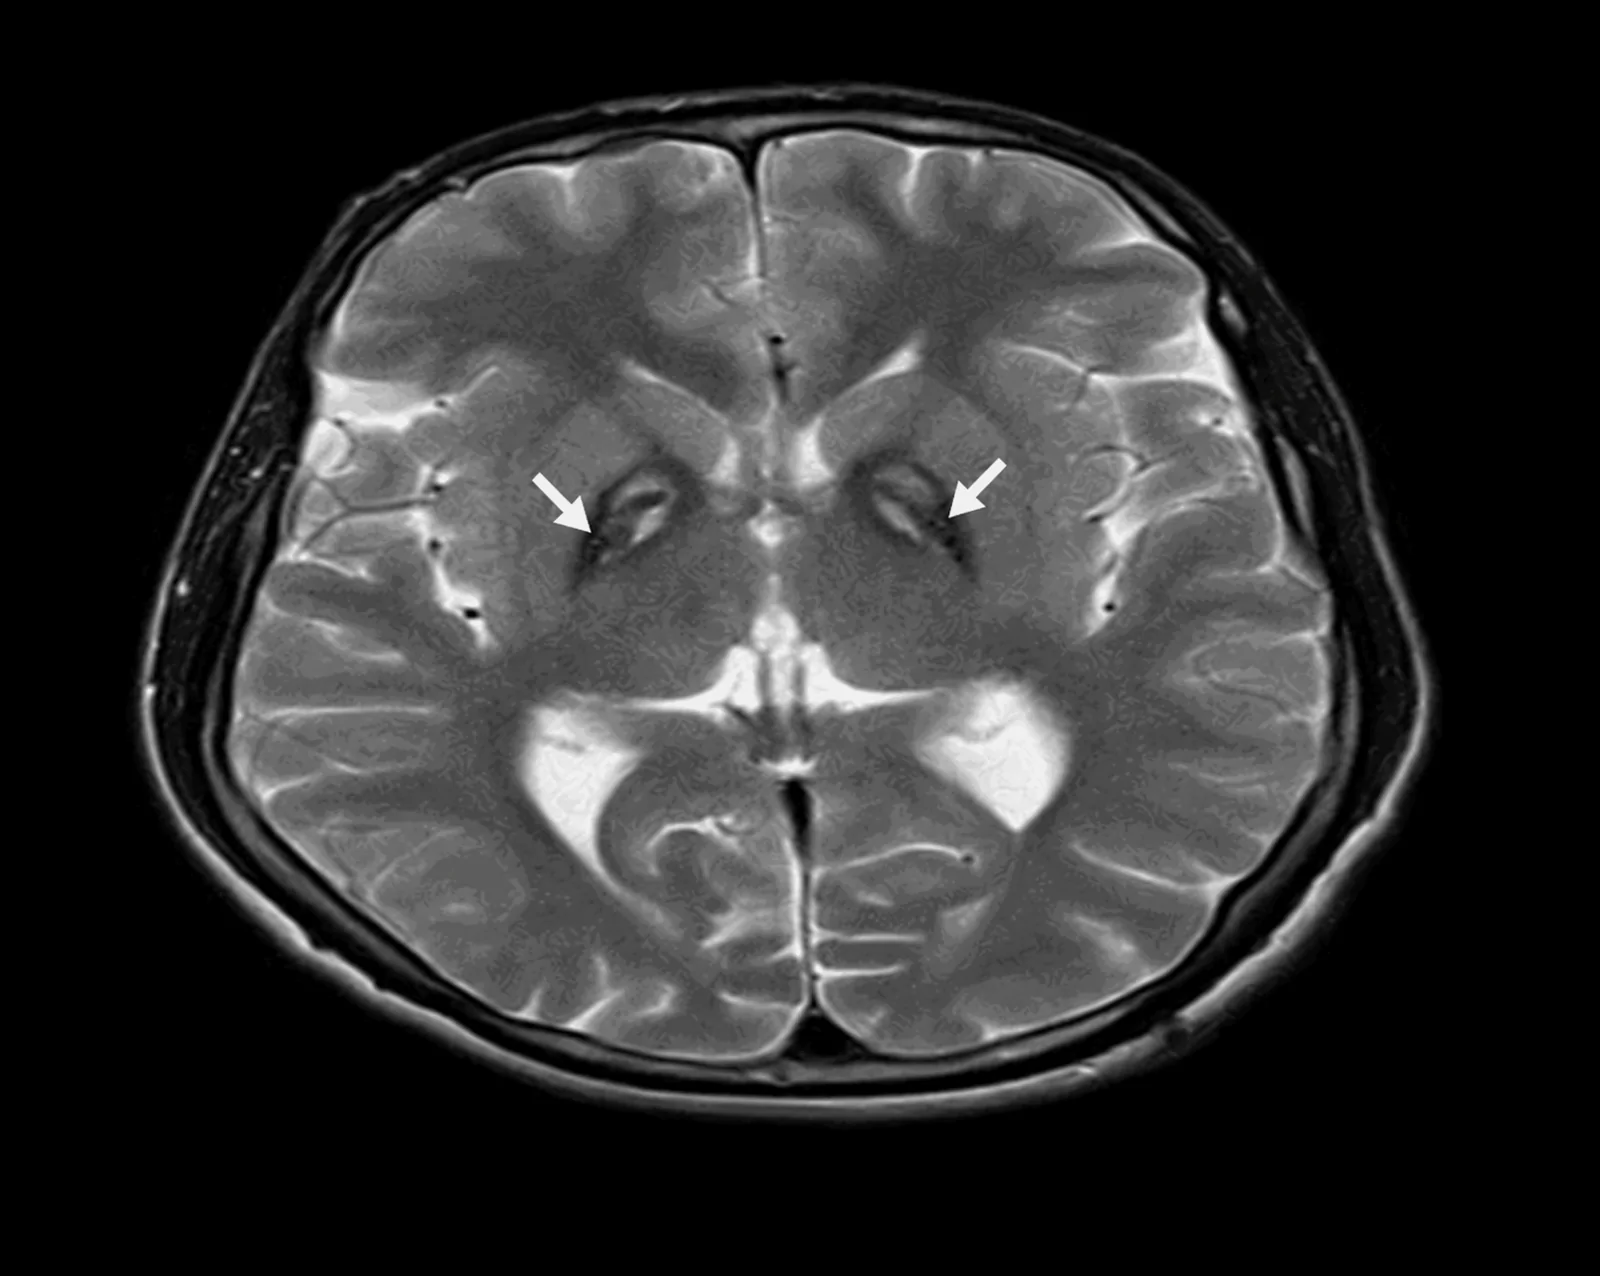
Axial T2-weighted MRI of the brain. Axial T2-weighted magnetic resonance imaging (MRI) of the brain demonstrates bilaterally symmetrical hypointensity in the medial globus pallidus (white arrows), suggestive of iron deposition. Notably, there is no central hyperintensity, and the classic *eye-of-the-tiger *sign is absent, indicating an atypical radiological presentation of pantothenate kinase-associated neurodegeneration (PKAN).
